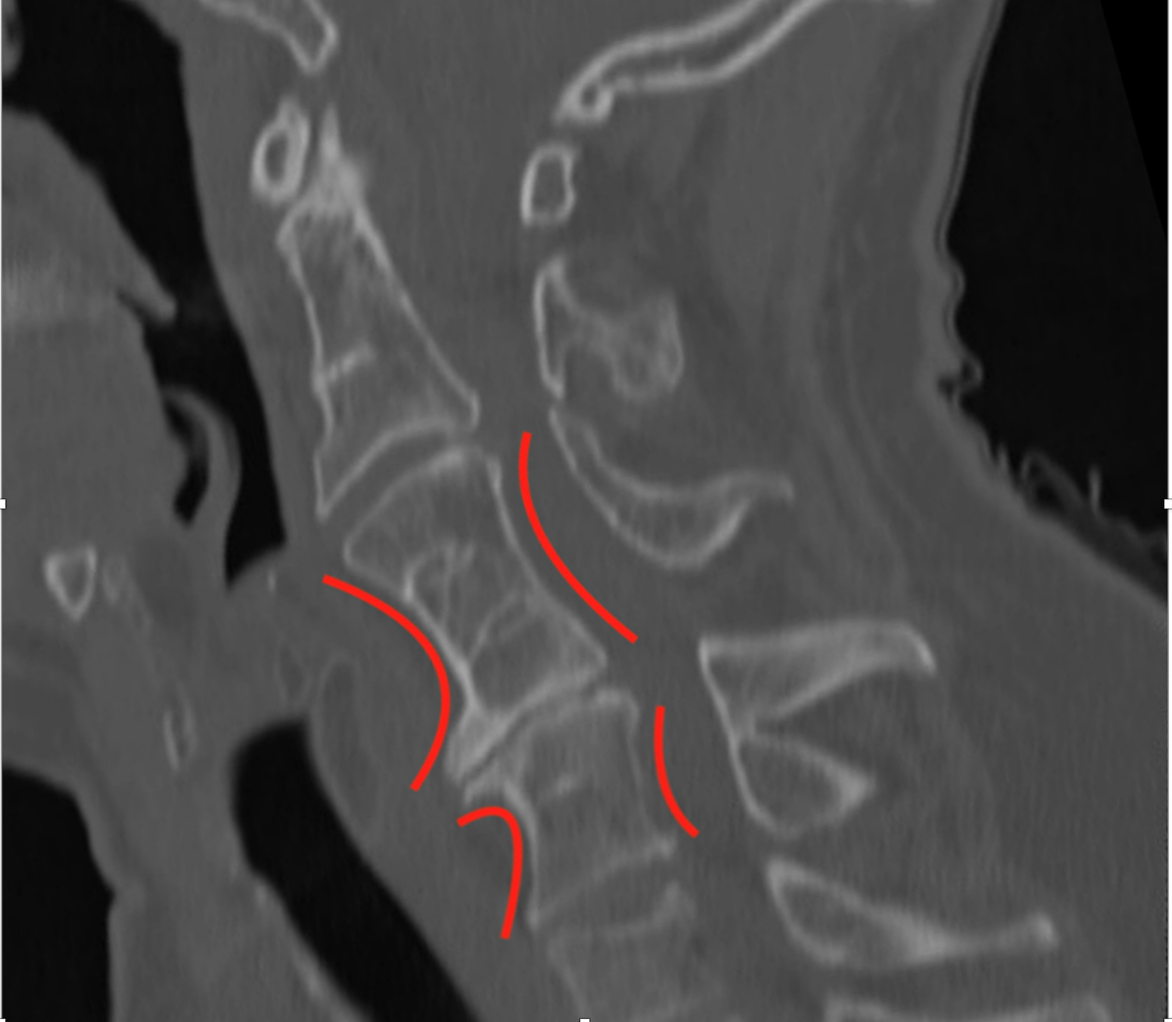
CT of Cervical Spine. A mid-sagittal reformatted CT scan of the cervical spine again showing fusion of multiple vertebral bodies, with associated kyphotic deformity of the cervical spine. Characteristic narrowing of the vertebral bodies, known as the Wasp-Waist sign (red curves) is also seen here.
